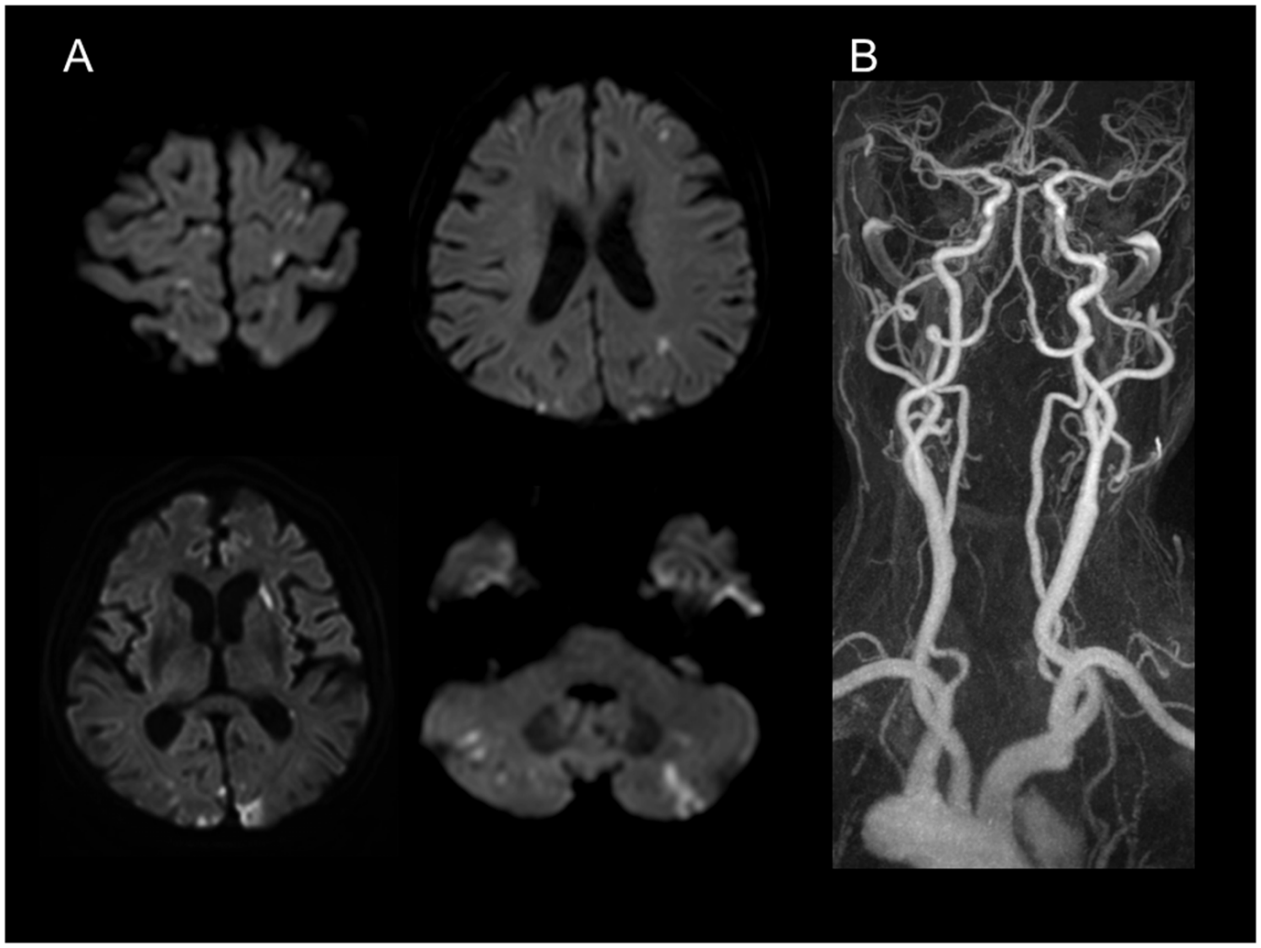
A typical example of cryptogenic stroke with active cancer (cancer-related stroke). The patient was a 55-year-old female, who was diagnosed with metastatic adenocarcinoma of lung one year ago. Neurologic examination revealed motor aphasia, right-central facial paralysis, and right hemiparesis. Initial D-dimer levels were 20.56 µg/mL. Diffusion-weighted MRI (A) shows multiple lesions involving multiple vascular territories. MR angiography (B) reveals no significant stenosis or occlusion of the craniocervical arterial vasculature. Comprehensive workup including transesophageal echocardiogram and 24-hour Holter monitoring demonstrated no sources of cardioembolism.
